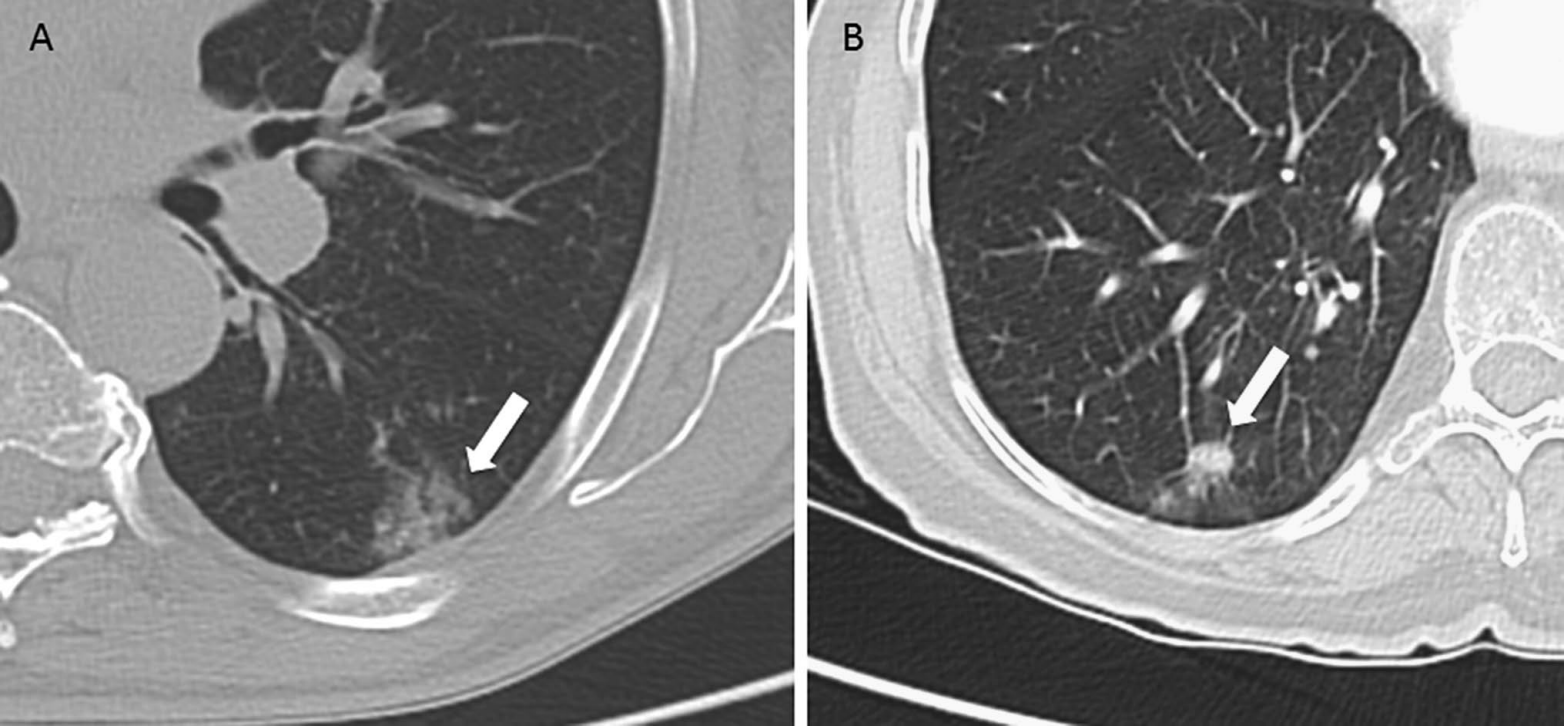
Role of thoracic CT in the characterization of pulmonary nodules. a 75 year-old male with a 2.5 cm subsolid nodule that experienced no change during 2 years of follow-up CT (arrow). The biopsy showed in situ adenocarcinoma. b Patient with a subsolid nodule that includes a solid component (arrow). Pathology exam showed an invasive adenocarcinoma
